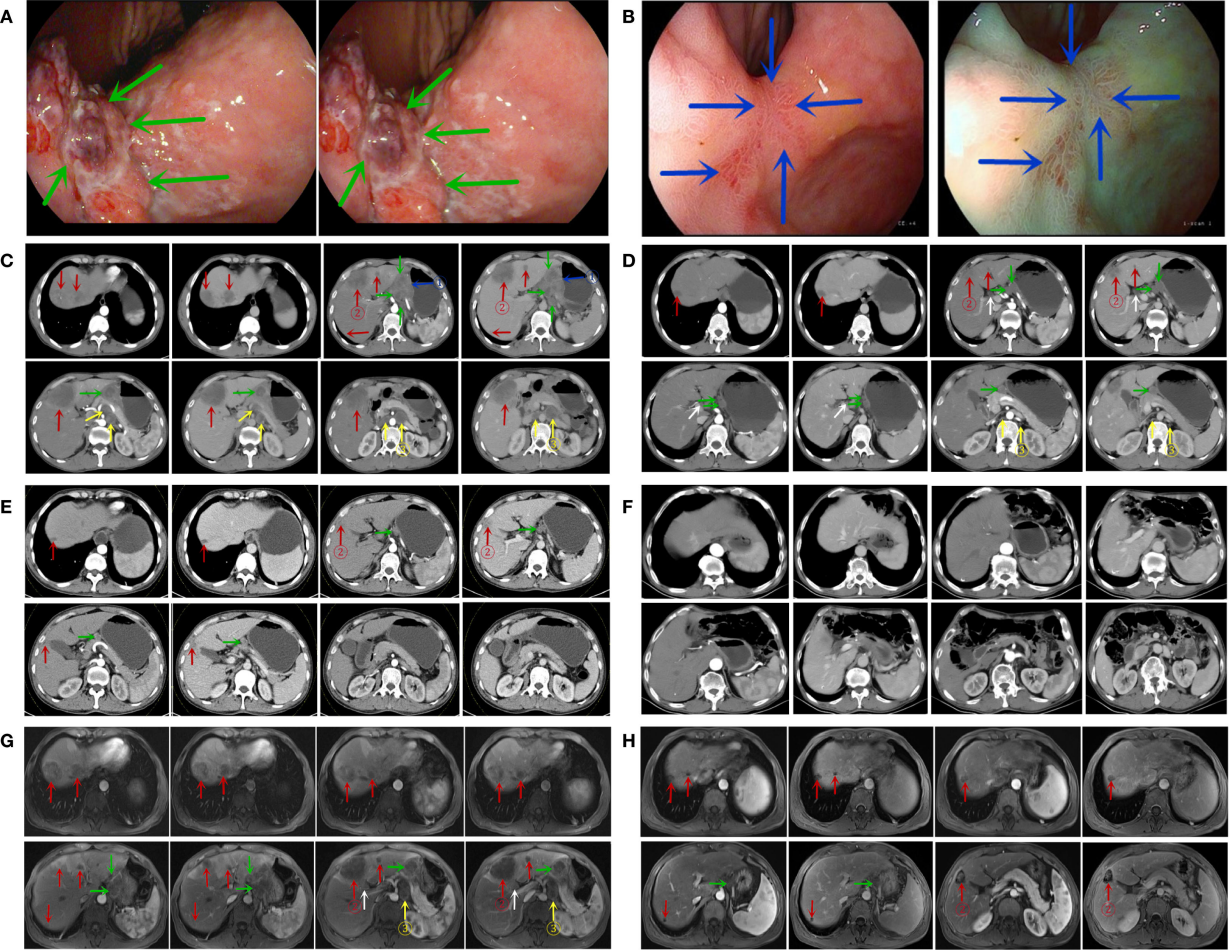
Gastroscopic findings (A, B). (A) Gastroscopy at the time of initial diagnosis; (B) gastroscopy at the conclusion of six cycles of treatment. (Notes: The green arrow indicates the lesion in the stomach prior to treatment, whereas the blue arrow indicates the lesion in the stomach at the conclusion of the 6-cycle treatment.) CT findings (C-F). (C) CT at the initial diagnosis (February 9, 2022); (D) CT at the conclusion of three treatment cycles (May 1, 2022); (E) CT at the end of six treatment cycles (July 27, 2022); (F) CT scan results from the most recent follow-up visit (April 26, 2025). MRI findings (G, H). (G) initial MRI at the time of diagnosis (February 9, 2022); (H) follow-up MRI after completion of six treatment cycles (July 27, 2022). (The blue arrows indicate the primary gastric lesion, the red arrows indicate liver metastases, the green arrows indicate perigastric lymph nodes, the white arrows indicate hilar lymph nodes, and the yellow arrows indicate retroperitoneal lymph nodes; ① represents the target lesion of the primary gastric lesion; ② represents the target lesion of the liver metastasis; ③ represents the target lesion of the retroperitoneal metastatic lymph node.).
